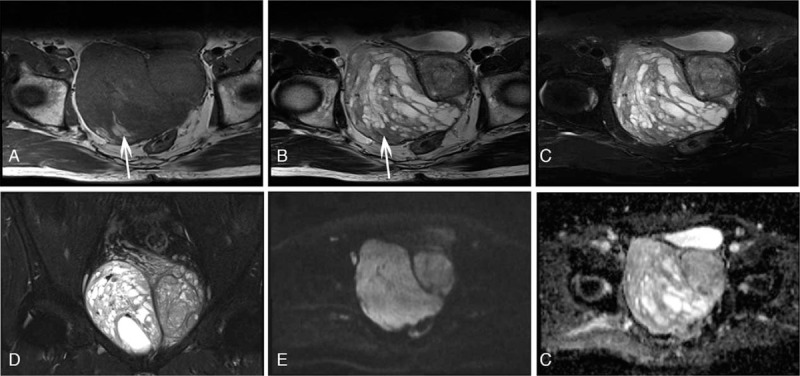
MRI features of the tumor. The tumor appeared as isointense on T1WI (A). The solid part of the tumor showed slightly higher SI on T2WI (B), and most of the cysts of the tumor showed hyperintense SI on T2WI. Some cysts that showed high SI on T1WI were hypointense on T2WI (arrow). C and D, T2WI fat-suppressed imaging showed the tumor was composed as a solid-cystic mass in the pelvic. The solid part of the tumor was slightly higher SI on DWI (b = 800) and ADC maps (E, F). ADC = apparent diffusion coefficient, DWI = diffusion-weighted imaging, MRI = magnetic resonance imaging, SI = signal intensity, T1WI = T1-weighted imaging, T2WI = T2-weighted imaging.
